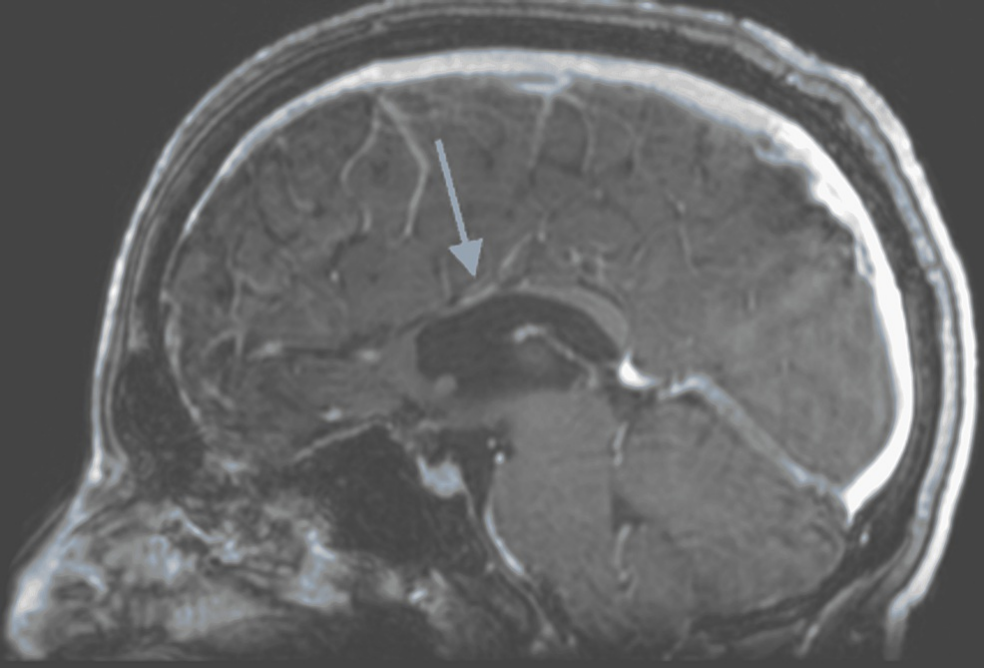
MRI head with an arrow identifying corpus callosum agenesis
